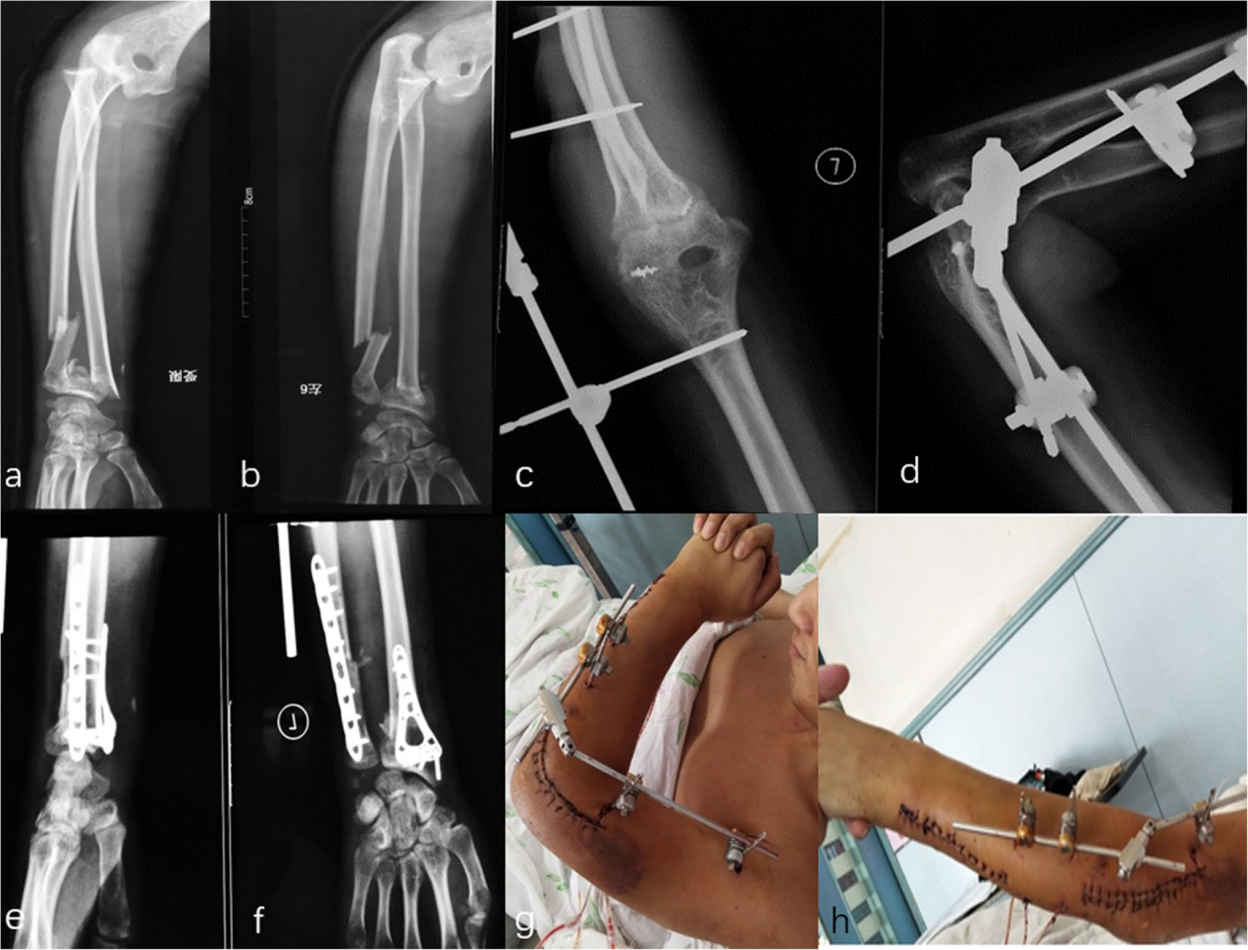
Anteroposterior and lateral radiographs revealed a DDE combined with distal ulna fracture, distal radius fracture and dislocation of DRUJ (a–b). Radiographic and clinical evidence demonstrated maintenance of the reduction of the PRUJ and DRUJ (c–h)
